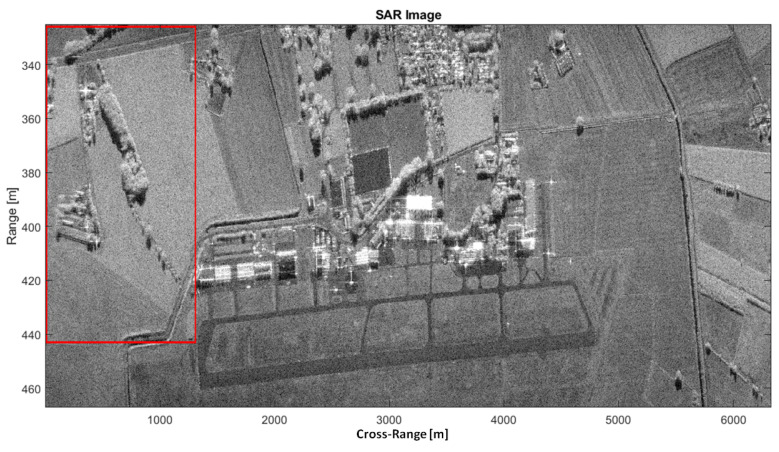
SAR image of the observed area formed via the range Doppler algorithm (RDA). The red box include the area of interest.
